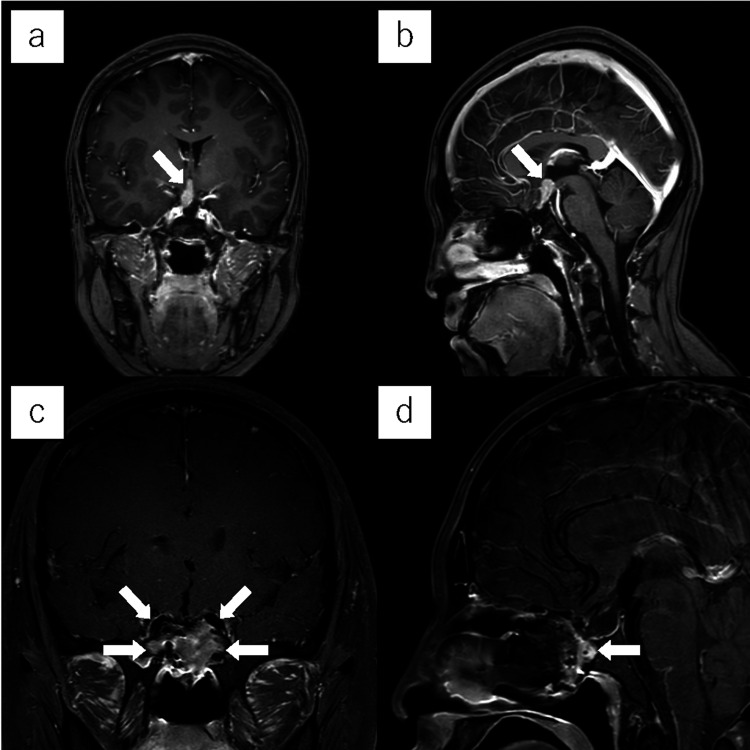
Pre- and postoperative contrast-enhanced MRI at the time of reoperation (a) Coronal and (b) sagittal contrast-enhanced magnetic resonance imaging before the fifth operation showed an enhancing 8 × 7 × 19 mm lesion to the right of the suprachiasmatic area extending to the third ventricle floor (white arrow). (c) Coronal and (d) sagittal images on the day after surgery showed complete tumor removal and sufficient enhancement of the posterior septal nasal floor flap (white arrows).
